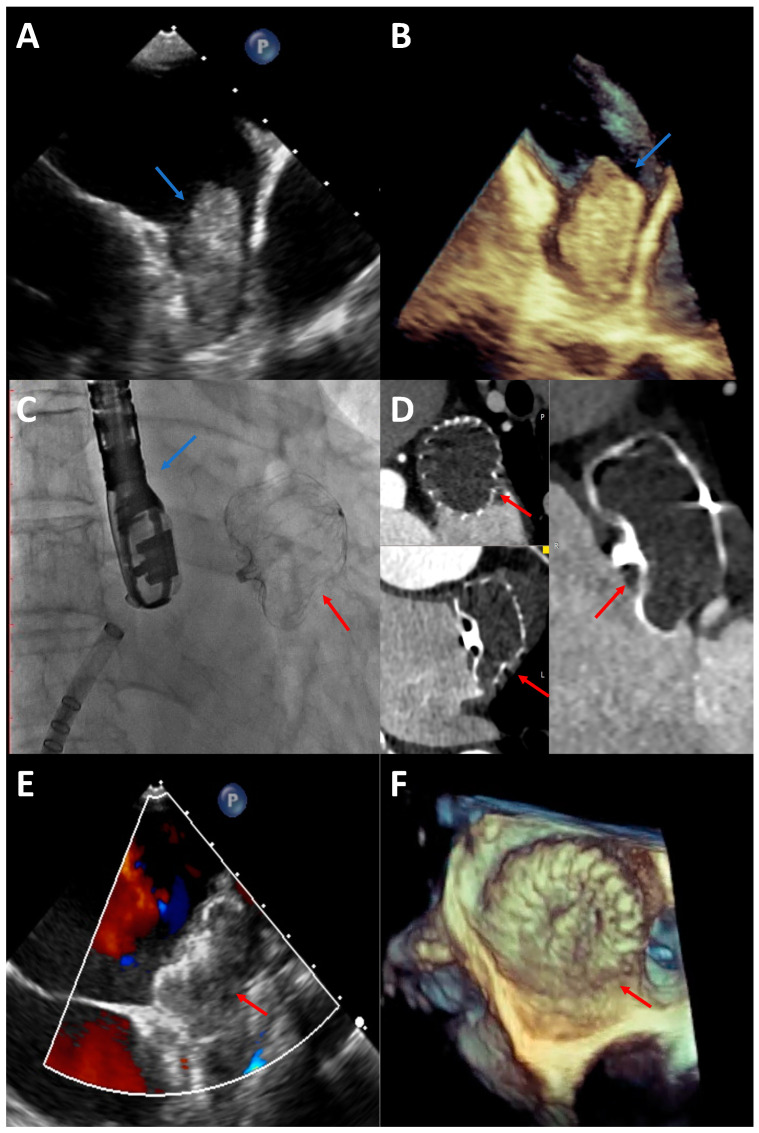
Imaging methods. (A) TOE—2D image. LAA with a thrombus (blue arrow). (B) TOE—3D image. LAA with a thrombus (blue arrow). (C) Intraoperative DSA. LAA occluder (red arrow) and TOE probe (blue arrow). (D) Follow-up CCT. LAA occluder (red arrows). (E) Follow-up TOE—2D image. LAA occluder (red arrow). (F) Follow-up TOE—3D image. LAA occluder (red arrow). Abbreviations: CCT—computer tomography; DSA—digital subtraction angiography; LAA—left atrial appendage; TOE—transoesophageal echocardiography.
